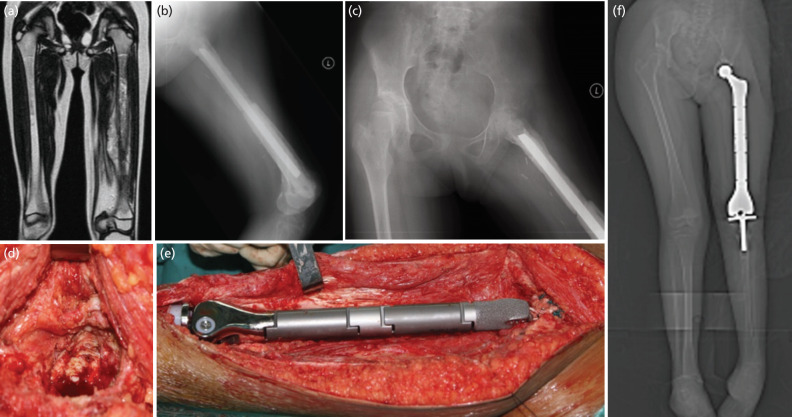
Case 6. A 8-year-old girl with diaphyseal osteosarcoma crossing the distal femur physis with bone marrow extension to the trochanter was initially managed with resection of the entire femur with allograft reconstruction. Four years later, the entire construct was converted to total femur endoprosthesis at her age of 12 due to severe acetabulum erosion and pain. The limb length discrepancy was managed by contralateral all physeal epiphysiodesis. She was ambulating pain-free without support with 3cm shortening 12 years following TFR surgery. (a) MRI showed extensive marrow involvement of the entire femur, (b) total femur allograft reconstruction, (c) acetabulum erosion on pelvic radiograph, (d) Operative photo showed acetabulum cartilage erosion, € Total femur endoprosthesis with hip replacement, (f) lower limb scanogram showed total femur replacement with limb shortening of 2cm.
